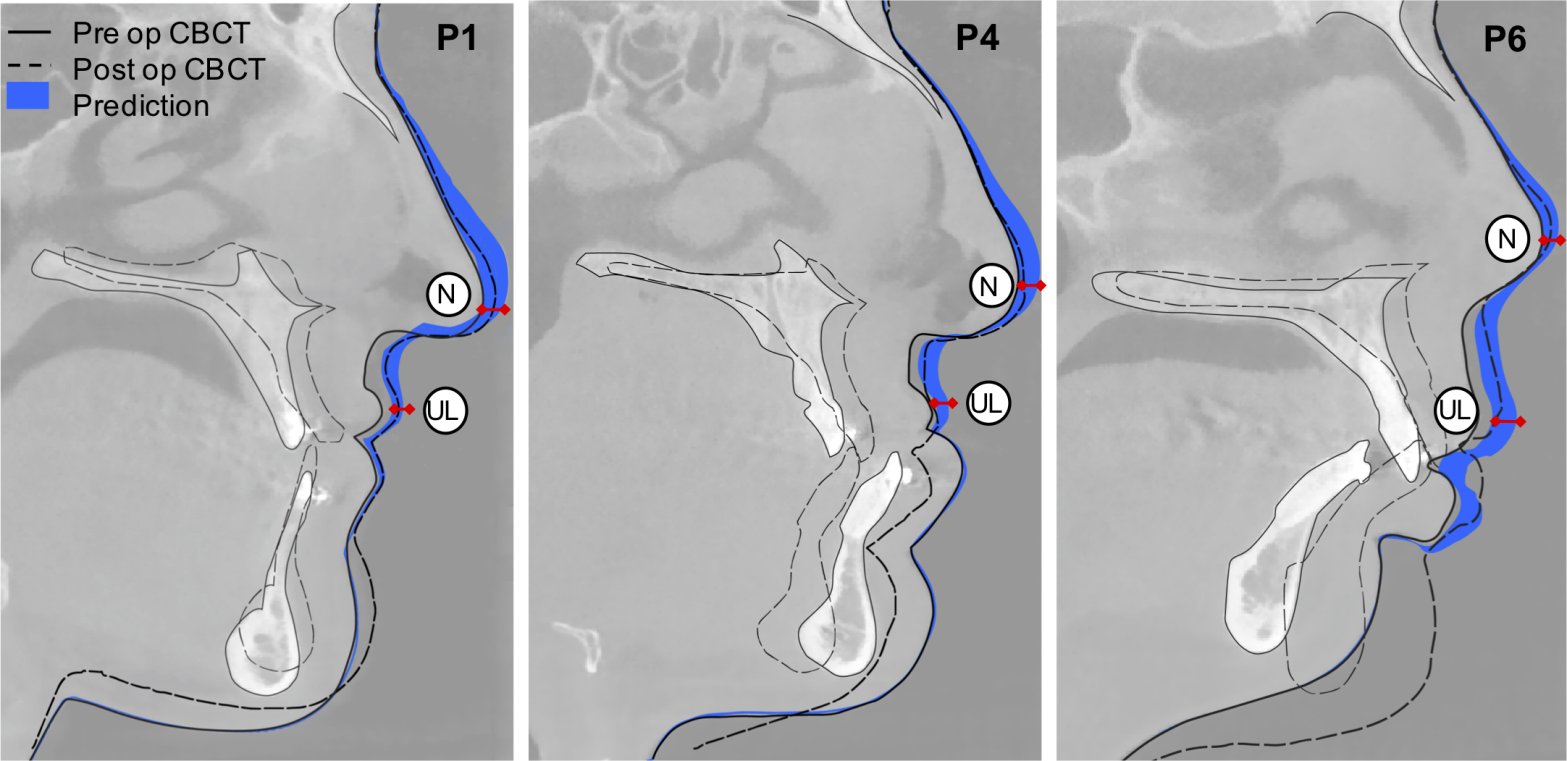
Midline 2D views for P1, P4, and P6. For each patient, preoperative CBCT (solid black line), postoperative CBCT (dashed line), and probabilistic soft tissue prediction (blue area) are shown, as well as indications for the nose (N), upper lip (UL), and lower lip (LL) points.
